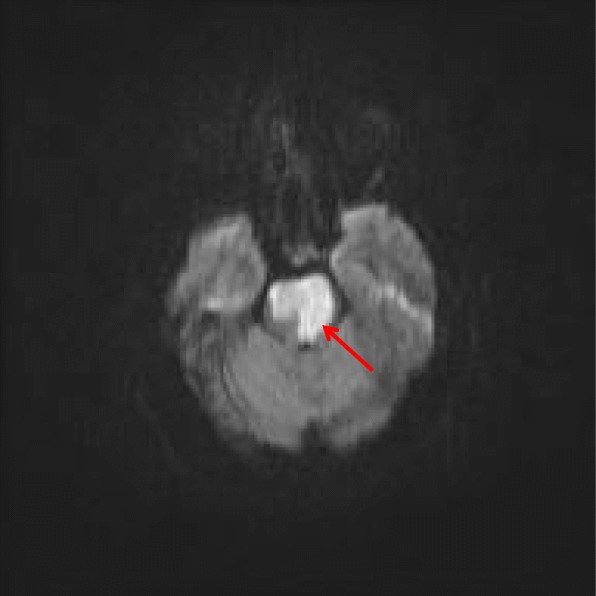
Magnetic resonance imaging of the patient’s brain without contrast from February 9, 2015. A large focus of abnormal restricted diffusion is demonstrated in the brainstem (*red arrow*), consistent with recent infarction. This primarily affects the cranial aspect of the pons, possibly extending into the midbrain. This is a bilateral finding, with more extensive involvement on the left. This is concordant with findings from computed tomography of the patient’s head on February 7, 2015. Mild associated mass effect and swelling are present at this time
